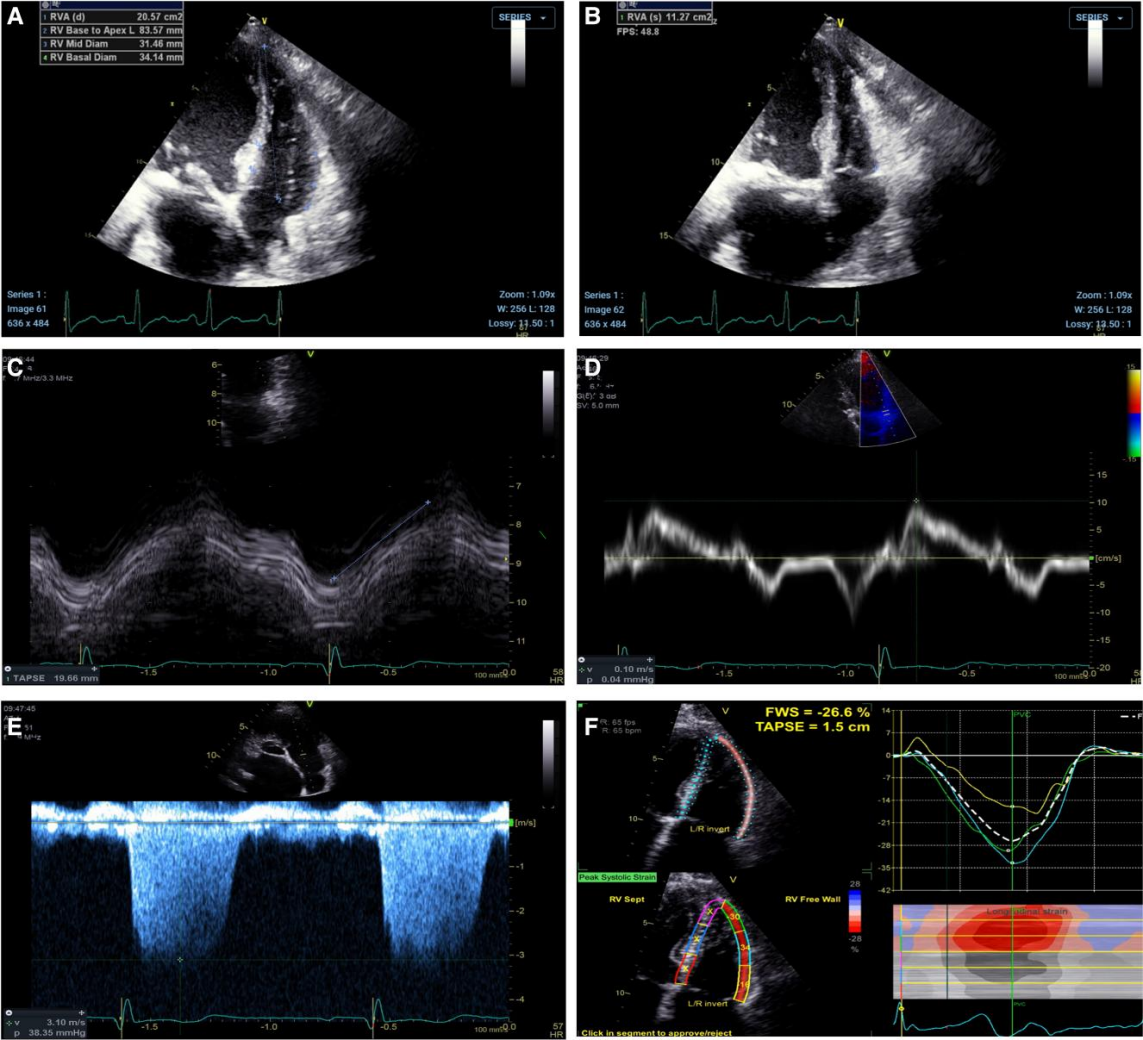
Representative echocardiographic images of the acquisition of right ventricular size and function parameters. A, end diastolic area; B, end systolic area; C, tricuspid annulus plane systolic excursion; D, S’; E, tricuspid regurgitation velocity; F, right ventricle free wall strain.
